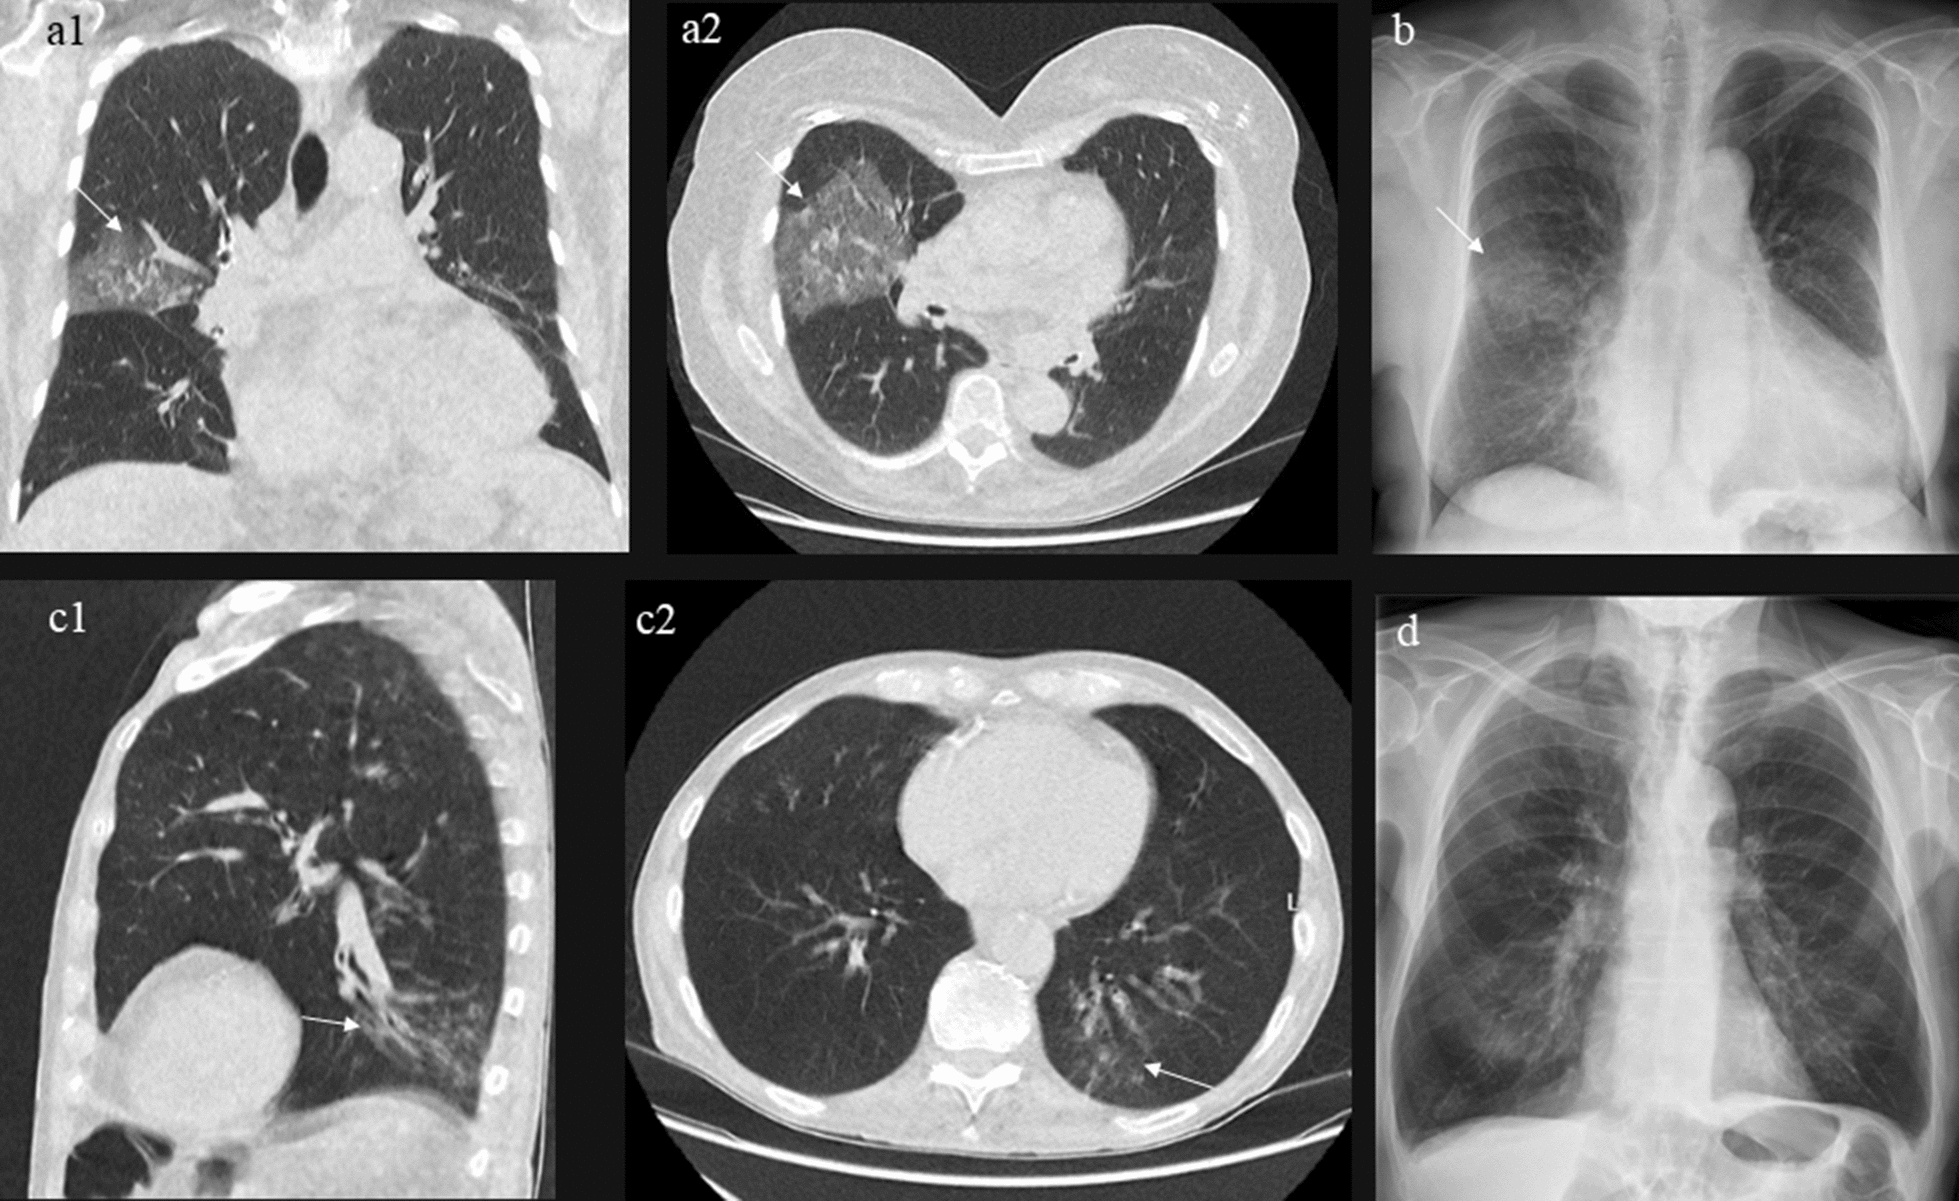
Examples of images from the study population (a) Ultralow-dose computed tomography (ULD-CT) of the chest from a patient with an opacity consistent with community-acquired pneumonia (CAP) in the right lobe, which is also seen in the corresponding chest radiograph (b). (c) ULD-CT of the chest from a patient with a left lower lobe opacity consistent with CAP. This opacity was not initially identified on the corresponding chest radiograph by the reporting radiologist (d). After reviewing the ULD-CT and comparing it with the chest radiograph, a small pneumonia could be suspected upon a second examination of the images (c, d)
